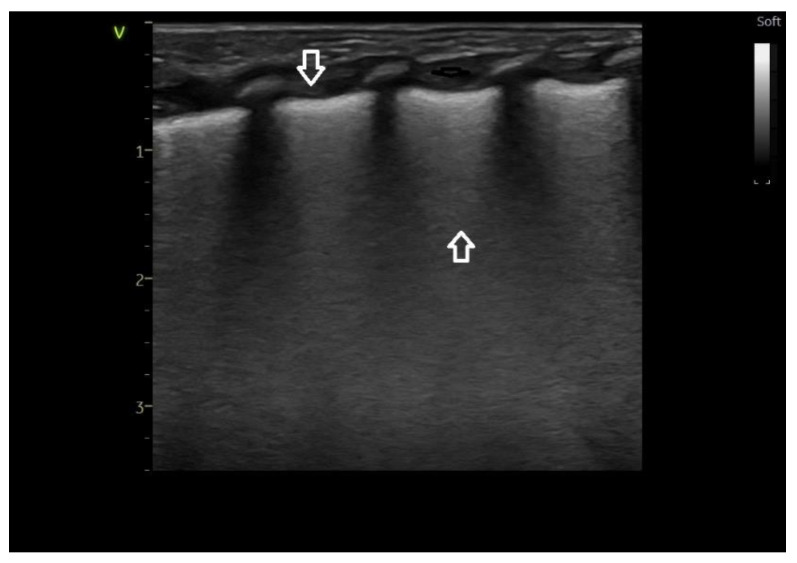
Lung ultrasound appearance of respiratory distress syndrome due to surfactant deficiency—white lung image, coalescent B-type lines occupying all lung fields—vertical arrow up; thickened pleural line–vertical downward arrow. Compare with Figure 1—normal lung.
